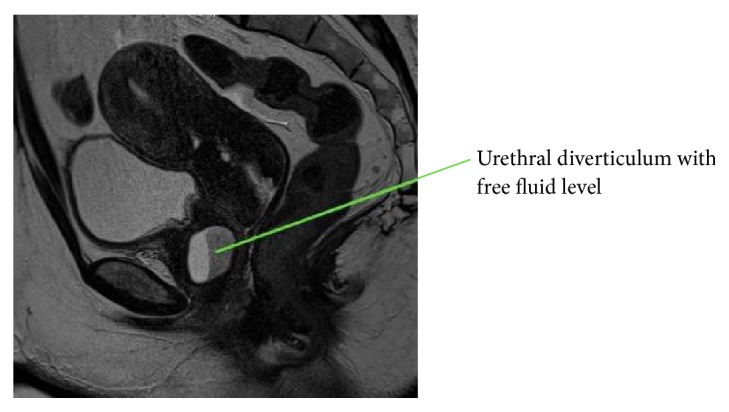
Pelvic MRI, sagittal view of urethral diverticulum.
